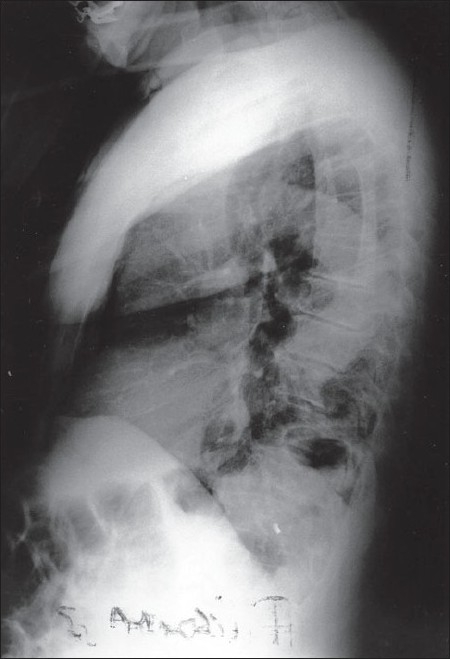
The chest X-ray on admission. L view
